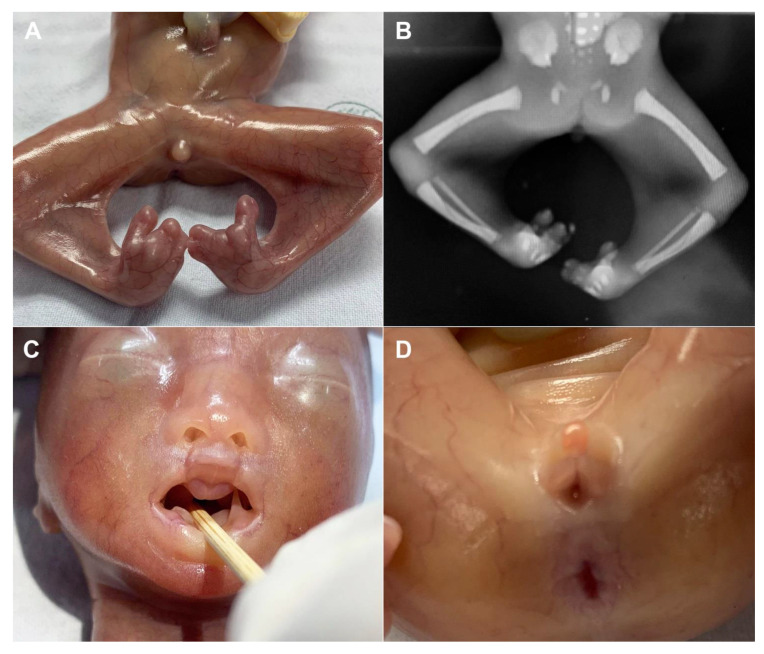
(A) Postnatal findings of the popliteal webbings and syndactyly/ectrodactyly; (B) X-ray of the lower limbs (abnormal postures); (C) Postnatal findings of the grooves in the upper lip and intraoral soft tissue adhesion; (C) Ambiguous genitalia (poorly developed scrotum with hypospadias).
